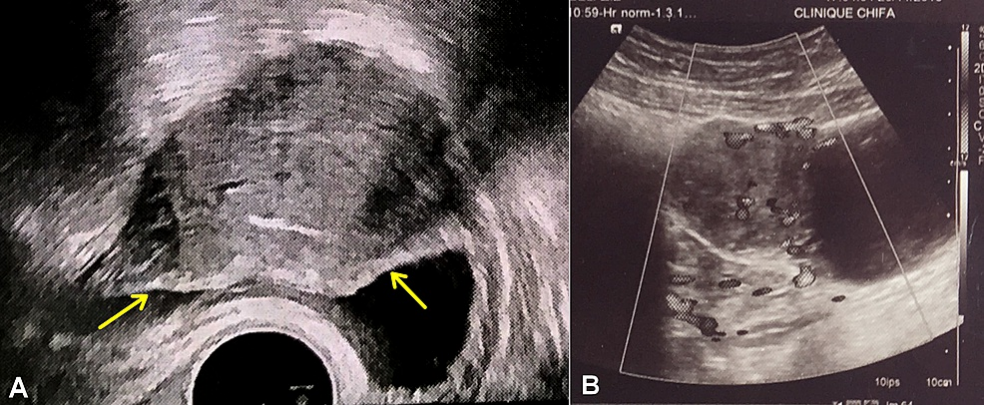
Ultrasound image of the urinary bladder showing an intramural mass along the dome of the bladder. (A) Axial TVS image showing a preserved epithelial lining (yellow arrows). (B) Sagittal transabdominal US image showing marked intralesional and peripheral flow signals on color Doppler.
